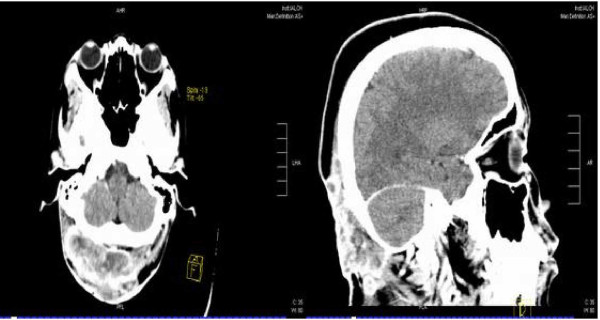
Enhancing mass lesion in occiput on axial and saggital CT slices.
